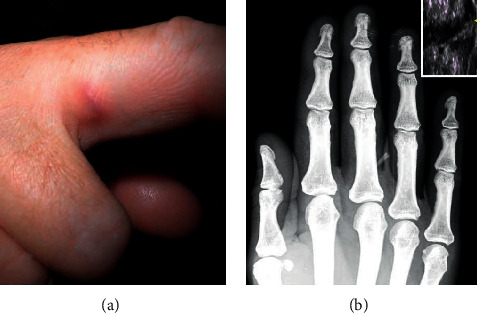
(a) Skin lesion at the 2nd admission. (b) X-ray film of the right hand demonstrating a wedge-formed radio-opaque foreign body in the soft tissue of the lateral aspect of the 1st phalanx of the middle finger. Inset: ultrasound detail of the foreign body insertion site.
